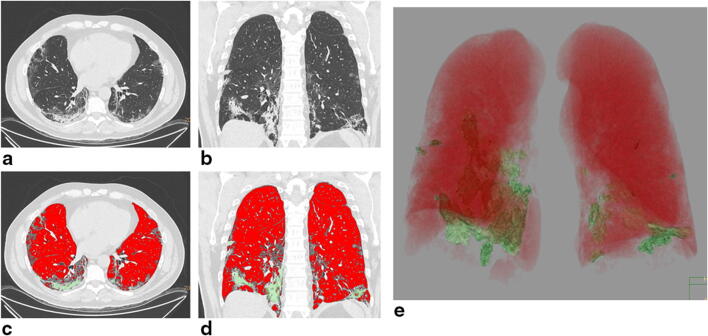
A 67-year-old man with SARS-CoV-2 related pneumonia. CT images in axial (a) and coronal (b) planes, showing bilateral multiple lobular ground-glass opacities associated with consolidations especially in the lower lobes. Final CT score: 24. Post-processed CT images in axial (c), coronal (d) sections and 3D reconstruction showing the ventilated lung volume (red colored), with an attenuation value lower than − 700 HU, and consolidation volumes (green colored) (e). The remaining volume is referred to as ground-glass opacities volume with a density between − 700 HU and − 350 HU
